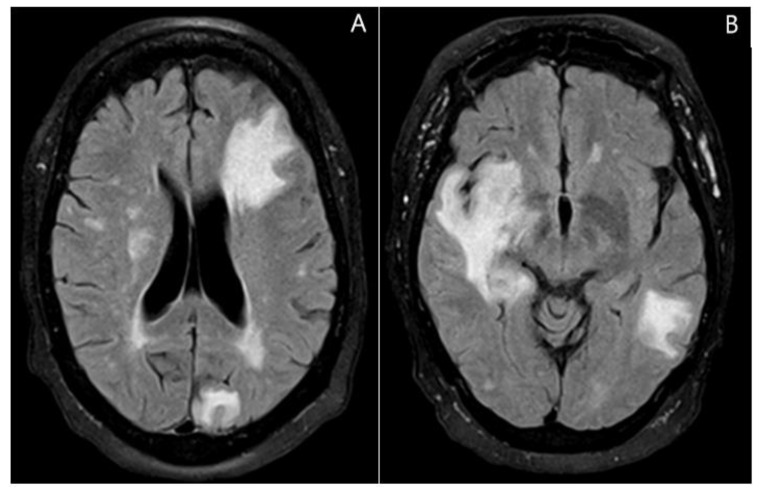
(A) Axial MRI FLAIR image demonstrating a hyperintense signal in the left frontal region left occipital and changes in a patchy manner occurring bilaterally. (B) There is a vasogenic edema and mass effect within the anterior right temporal lobe and a posterior left temporal lobe involving the left temporal stem and mesial temporal lobe.
